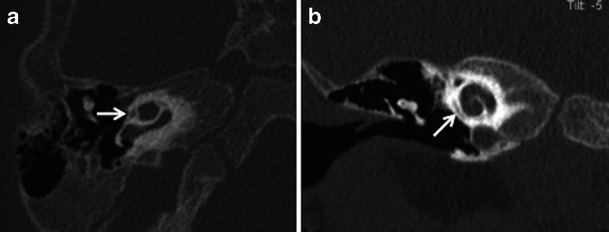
Axial (a) and coronal (b) HRCT images of the right temporal bone in a child with suspected left SNHL. The small lucency seen around the cochlea (*arrow*) on both the axial and coronal images is in keeping with a cochlear cleft (normal variant)
